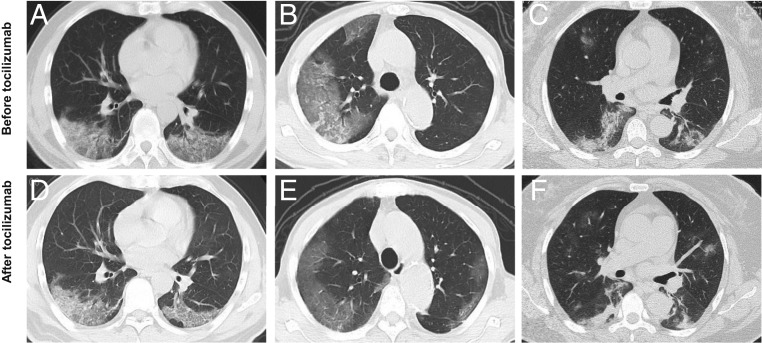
Chest CT scans showed significant remission in both lungs in patients after the treatment with tocilizumab. (*A*–*C*) Computerized tomography (CT) showed plaque-like and ground glass opacities before the treatment with tocilizumab. (*D*–*F*) Chest CT showed diffuse infiltration in both lungs, but the lesions were clearly absorbed after the treatment with tocilizumab.
